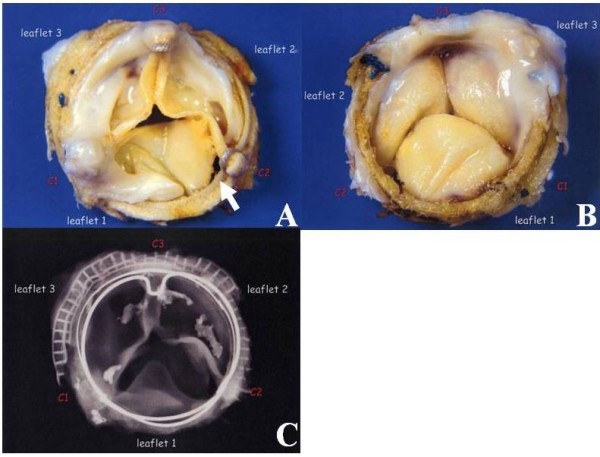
Explanted Carpentier-Edwards Perimount bioprosthesis (view from the left ventricule: A, view from the left atrium: B showing a tear in leaflet 1 (white arrow). X-ray of the valve showed calcification on leaflets 2 and 3, but not on leaflet 1 (C).
